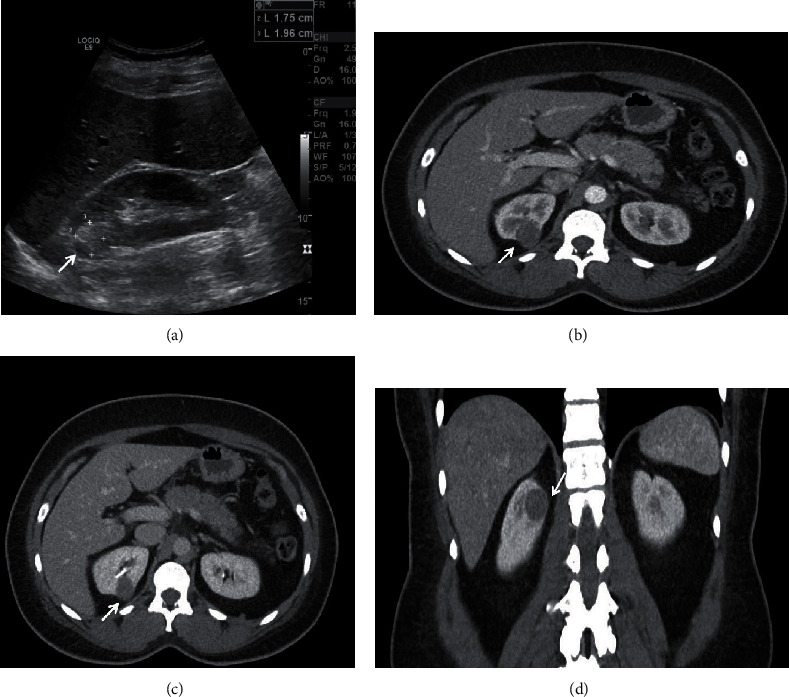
Patient preoperative imaging revealing the renal mass (white arrows). (a) Sagittal ultrasound image of the echogenic, solid renal mass measuring 1.75 cm by 1.96 cm. (b, c) Axial CT images with contrast highlighting the posterior location of the mass, its enhancing posterior wall, and nearness to the collecting system. (d) Coronal CT image of the same mass and its upper pole location. No calcifications were noted throughout the mass.
